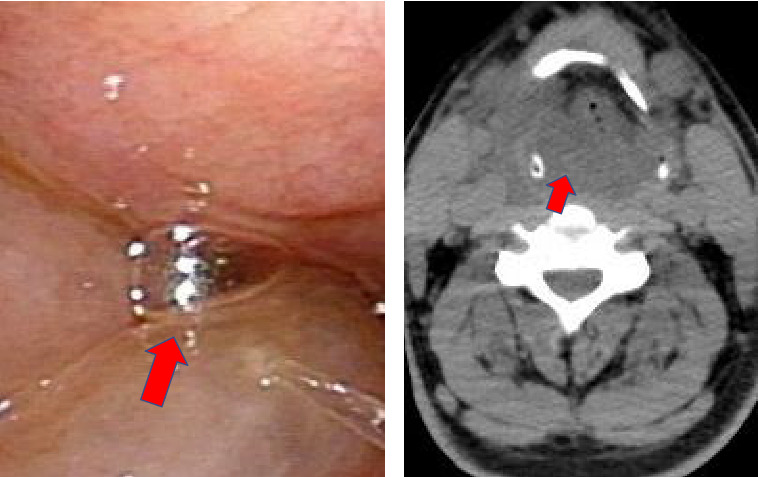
Laryngoscopy (a) and computed tomography (b) on the admission of the patient, a previously healthy 44-year-old male. His larynx was surprisingly swollen (red arrows).
